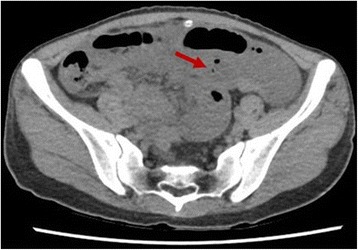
CT abdomen showing dilated bowel loops with transition point (arrow) in pelvis
